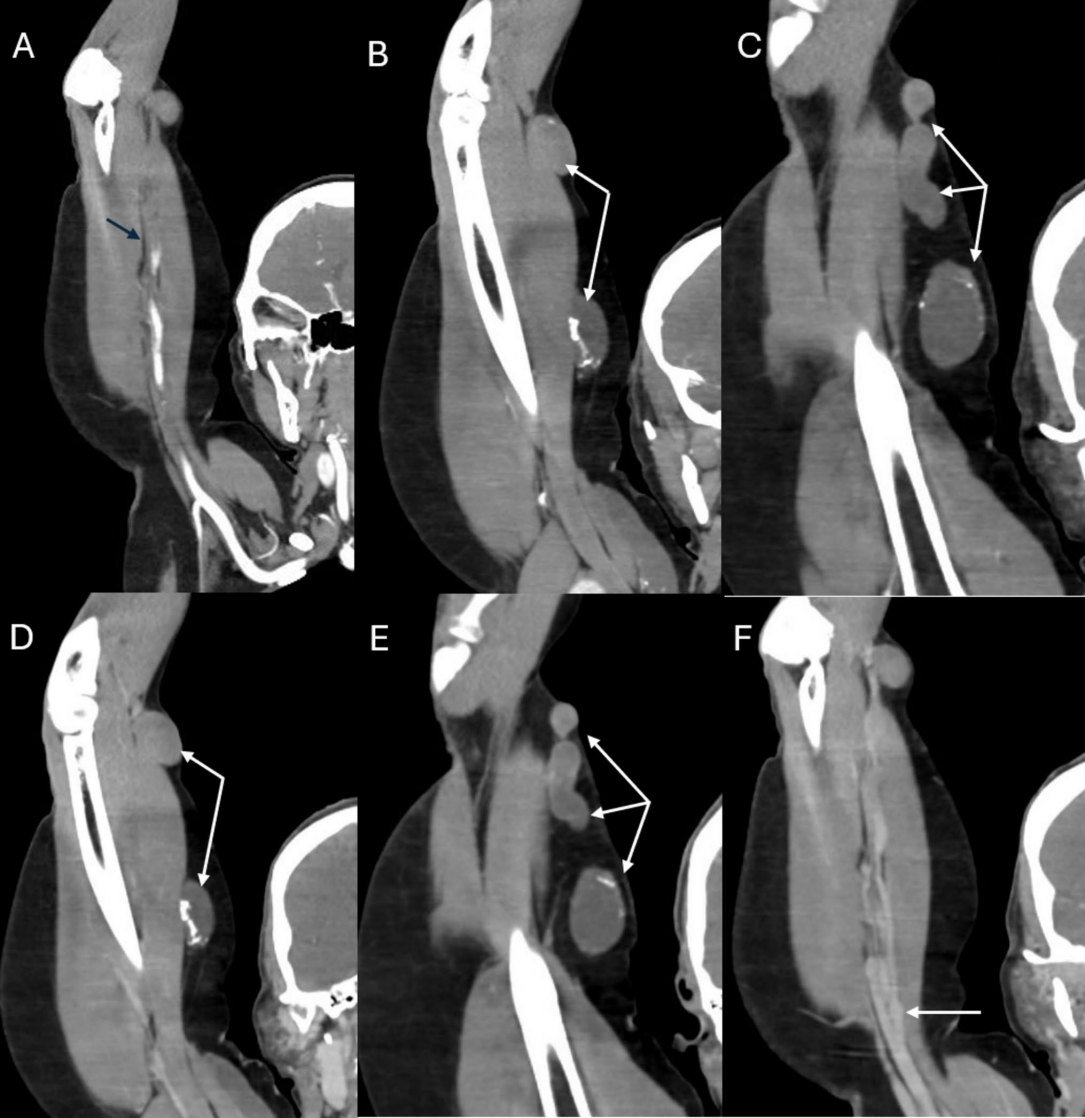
A 61 year-old female with right upper extremity brachiocephalic fistula for hemodialysis who presented with acute upper limb ischemia and therefore computed tomography angiography was performed. Arterial phase coronal reconstruction imaging showed a thrombosed brachial artery (black arrow in A). There is lack of opacification of the fistula (white arrows) on arterial phase images (B and C) as well as venous phase images (D and E). Patent venous outflow system as seen on venous phase coronal image (white arrow in F)
